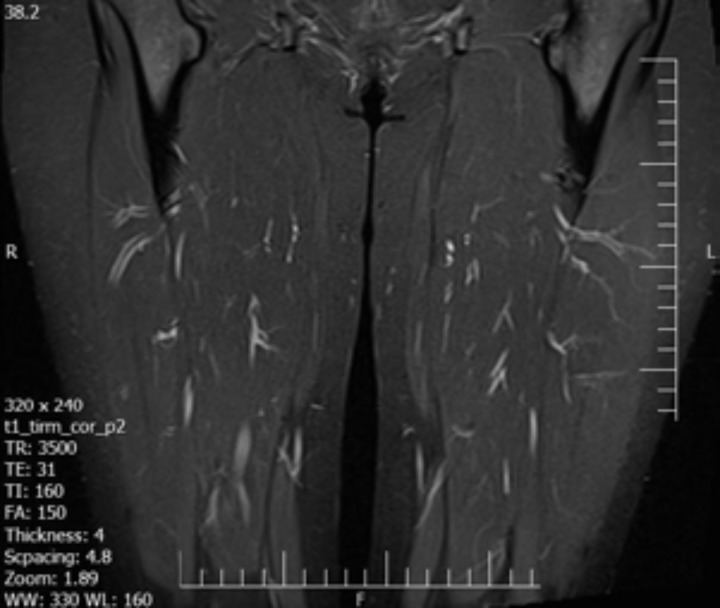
Muscle MRI showed no abnormal signal
